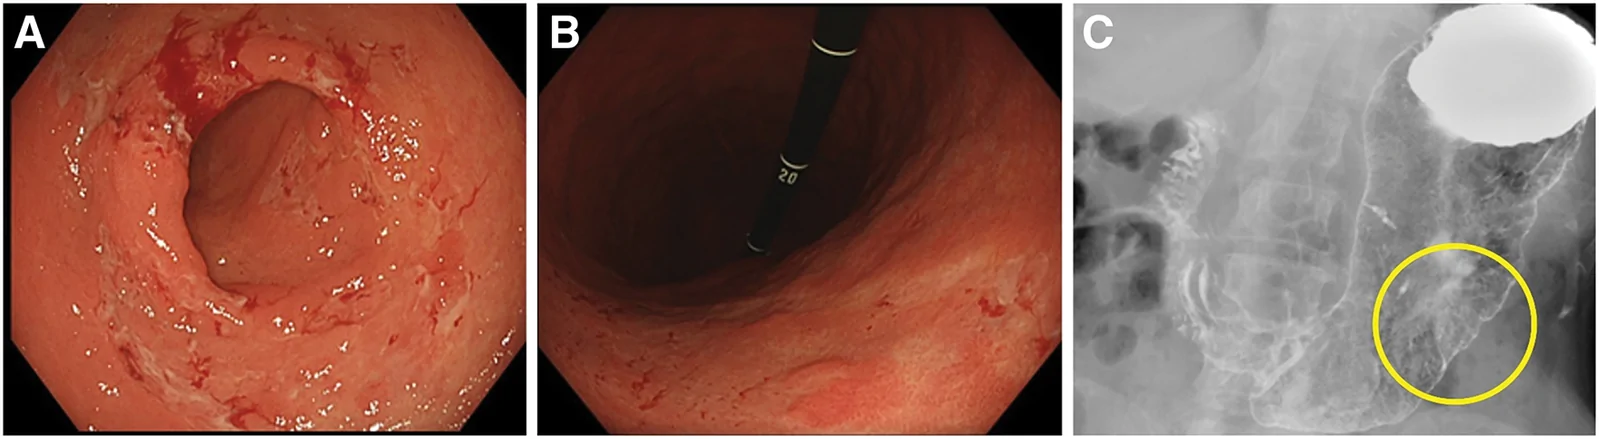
Endoscopic and radiographic findings of gastric cancer. (A, B) Upper gastrointestinal endoscopy showing an irregular ulcerative lesion extending from the lower gastric body to the antrum. (C) Upper gastrointestinal contrast study demonstrating an irregular lesion at the same site (yellow circle).
